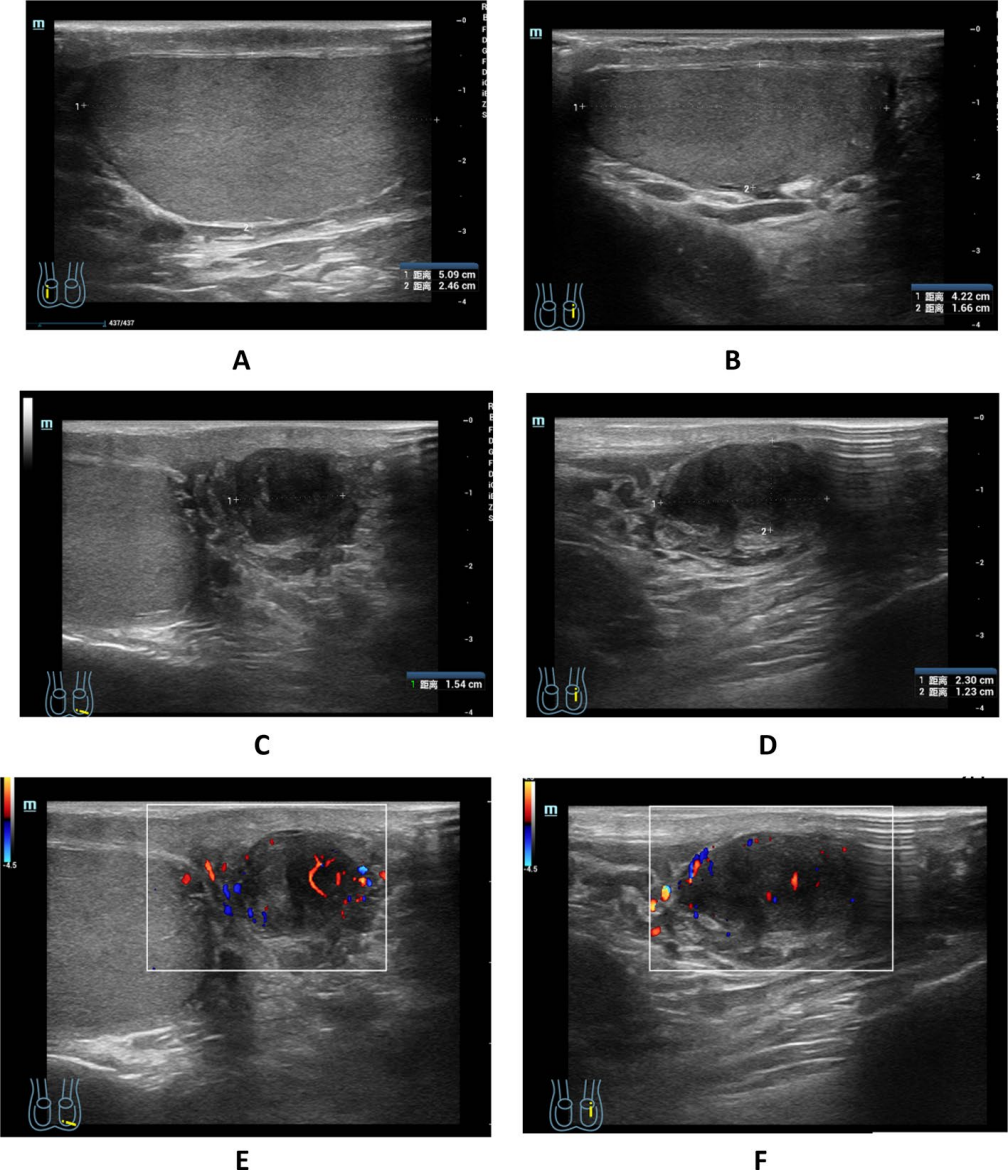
Color Doppler Ultrasonography images of the Epididymal leiomyosarcoma; Normal bilateral testes (A, B). A low-echoic nodule, approximately 2.30 × 1.23 × 1.54 cm, was identified in the tail of the left epididymis (C, D), Blood flow signals were detected (E, F)
